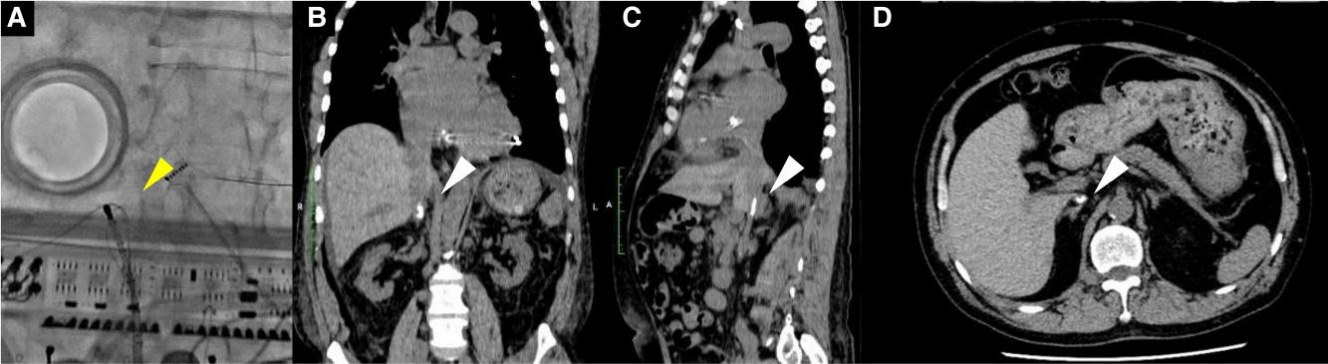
Fluoroscopy (*A*) and computed tomography (*B–D*) revealed that both the radiofrequency ablation catheter and the 0.035 in spring coil wire could not advance from the femoral vein to the right atrium due to calcified venous thrombosis blocking the inferior vena cava at the renal vein level. The white arrowhead indicates the radiofrequency ablation catheter and the 0.035 in spring coil wire, while the white arrowhead shows calcified venous thrombosis.
